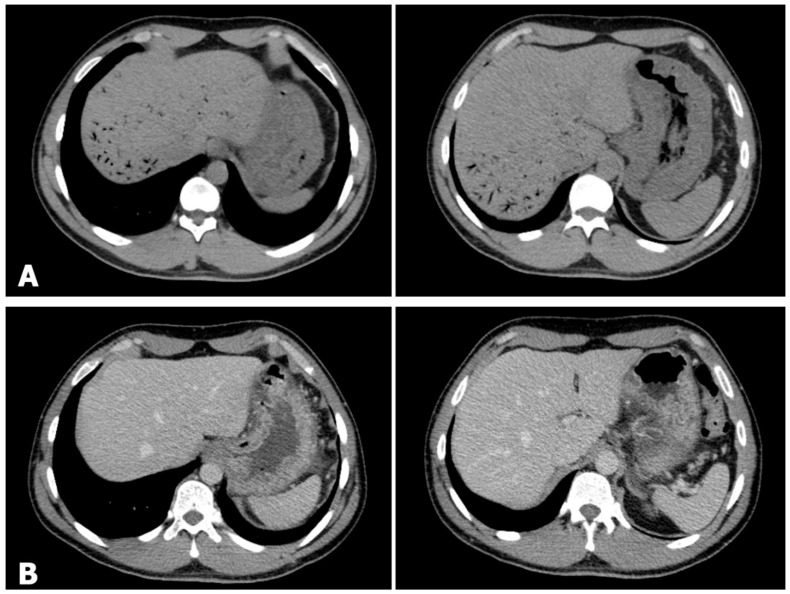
Considering the patient’s favorable clinical status and the absence of any radiological signs of perforation, a conservative approach was chosen. The patient was maintained on a fasting regimen and intravenous fluids and proton pump inhibitors were administered. On the day following admission, a comprehensive clinical assessment, encompassing a thorough examination of the abdomen and neurological function, yielded normal results. An upper endoscopy was also performed, confirming caustic agent damage of the gastric mucosa (Figure 4). Subsequently, a follow-up abdominal CT scan performed after the administration of intravenous contrast medium was carried out to re-evaluate the initial imaging observations (A). The follow-up scan (B) showed the spontaneous resolution of hepatic portal venous gas (HPVG) and the ongoing presence of the previously noted gastric and duodenal wall abnormalities.
